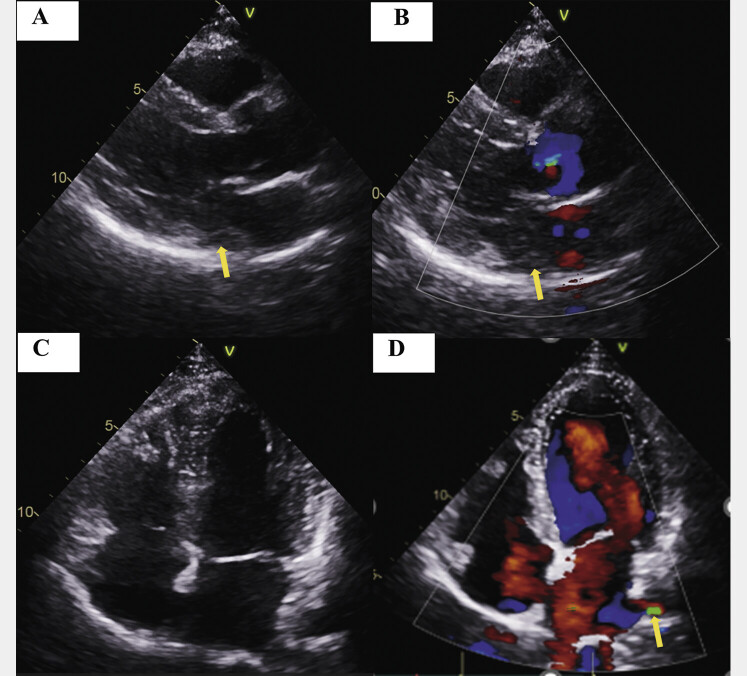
Transthoracic echocardiogram showed an occupying lesion in the pulmonary vein which extended into the left atrium.
